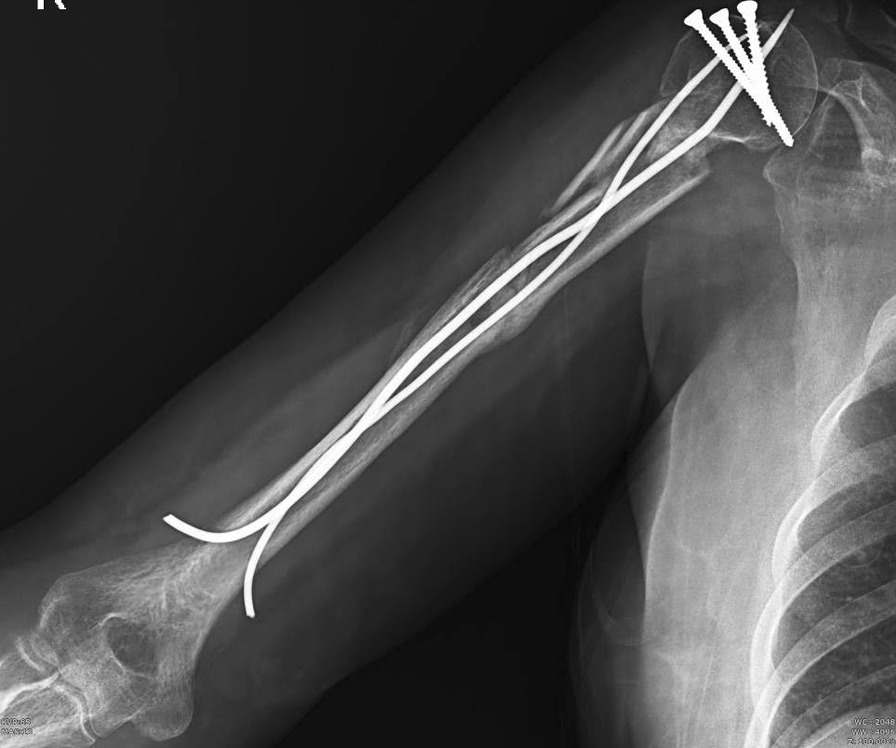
Anteroposterior X-ray showing partial union in second postoperative month
